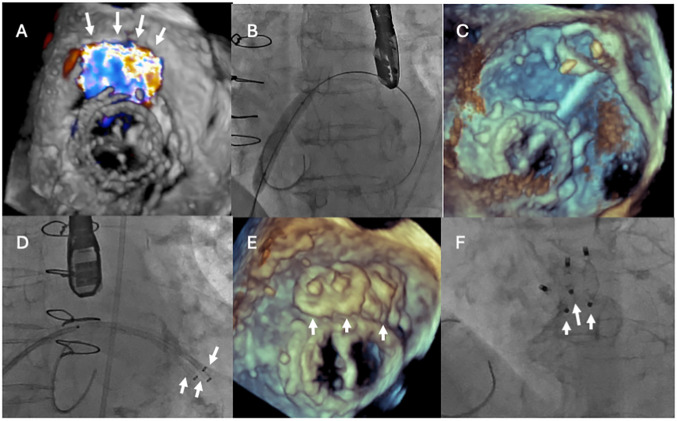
Representative case of anterior mitral paravalvular leak (PVL) closure guided by real-time transesophageal echocardiography (TEE) and fluoroscopy. White arrows indicate the paravalvular leak, delivery sheaths, or deployed occluder devices, as appropriate in each panel. (A) Pre-procedural 3D TEE showing a broad anterior mitral PVL (white arrows). (B) After transseptal puncture, the atrial septum was dilated with a balloon to facilitate catheter passage (not through the PVL orifice). (C) 3D TEE demonstrating advancement of the delivery sheath through the PVL orifice. (D) Fluoroscopic image showing three delivery sheaths crossing the defect (white arrows). (E) En-face 3D TEE view showing deployment of three Amplatzer Vascular Plug II (AVP II) devices (white arrows) at the anterior aspect of the sewing ring. (F) Final fluoroscopic frame confirming stable position of the three devices without interference
